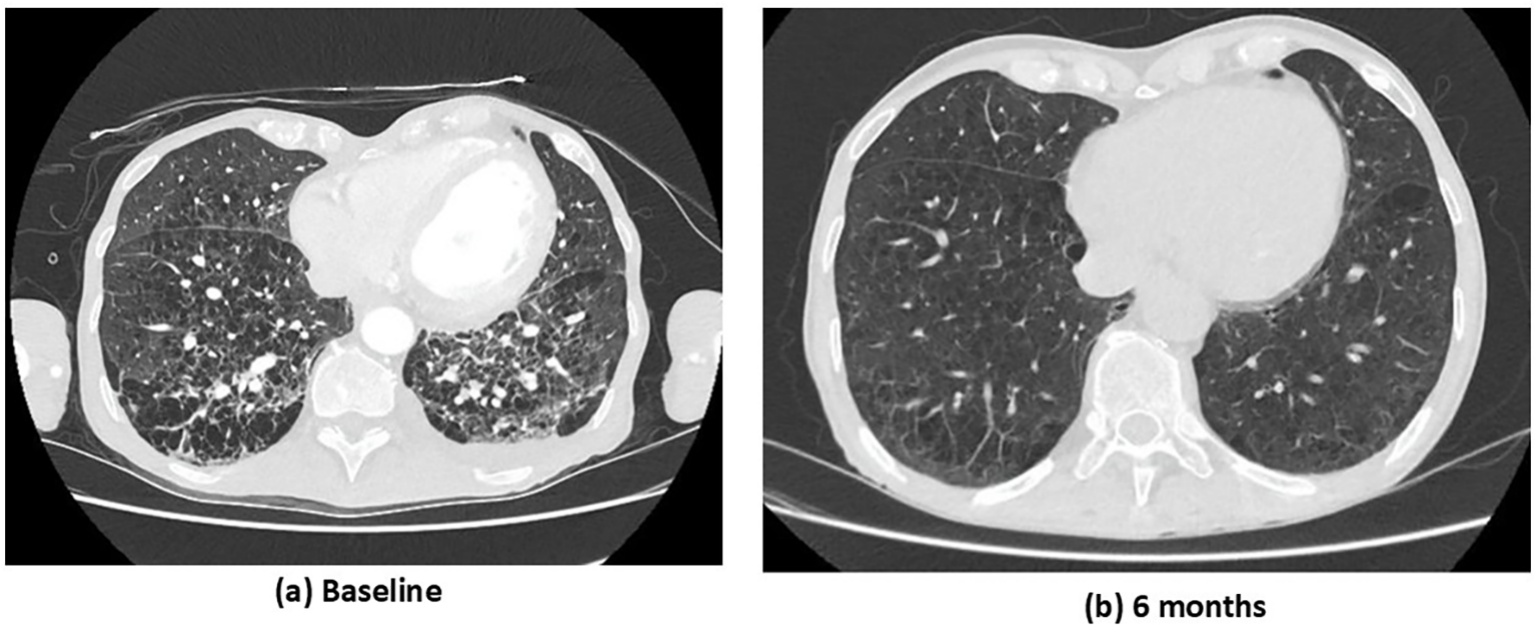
Case 1. CT chest study at baseline (a) and at 6 months following immunosuppressive treatment (b). (a) Baseline: extensive bilateral ground-glass opacities and interlobular septal thickening, multiple areas of consolidation and reticular changes, and honeycombing in the lower lobes. (b) Six months: marked radiological improvement with near-complete resolution of ground-glass opacities and consolidations, significant reduction in interlobular septal thickening and reticular changes, and minimal residual reticulation with subtle parenchymal changes.
